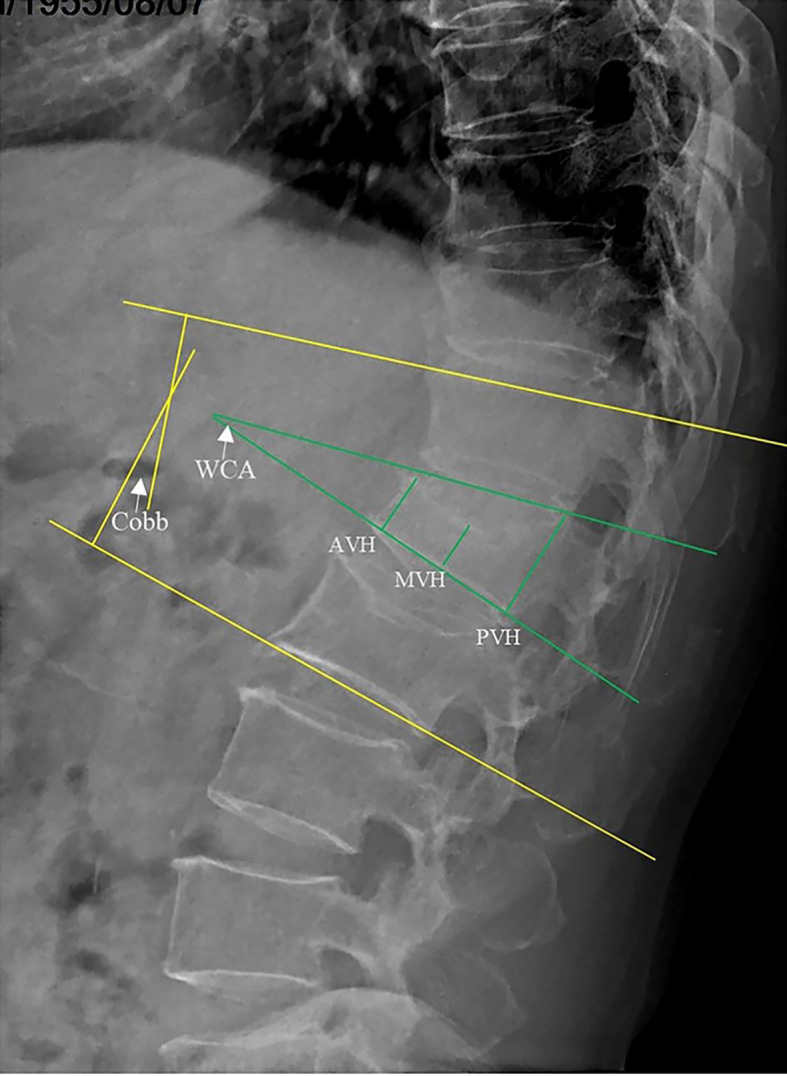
Radiographic measurements on lateral X-ray: Anterior vertebral height (AVH), middle vertebral height (MVH), posterior vertebral height (PVH), Cobb’s angle (Cobb), and wedge-shaped Cobb’s angle (WCA).
